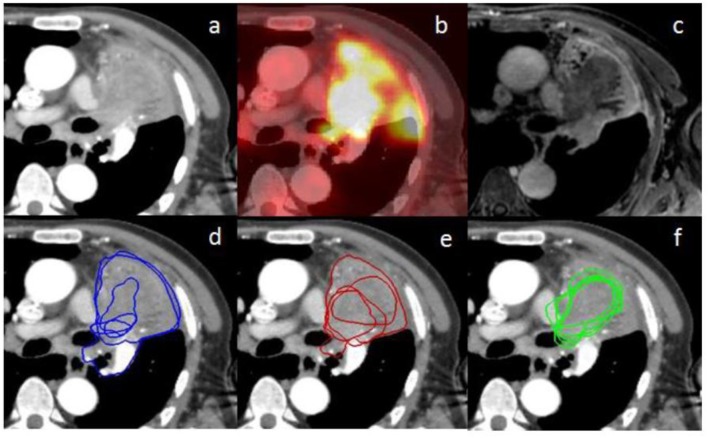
(a) represents an axial planning CT scan on the mediastinal window (+20/400 HU); (b) represents the fused PET scan corresponding to the planning CT scan (a) on rigid registration; (c) represents the MRI weighted T1 Lava Flex Water sequence at a delayed phase (8 min) corresponding to the planning CT scan (a) on rigid registration; (d–f) represent the contours from the five different observers, respectively, at the CT phase, PET phase, and MRI phase. There are only four visible contours for the PET phase since one of the volumes is outside, up to the CT scan. Inter-observer variability is lower for the MRI phase compared to the other phases.
